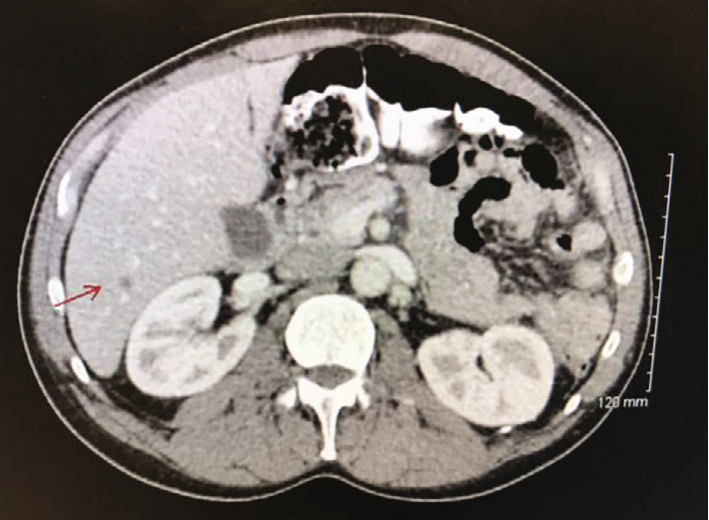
CT with contrast imaging of liver metastasis (arrow).
